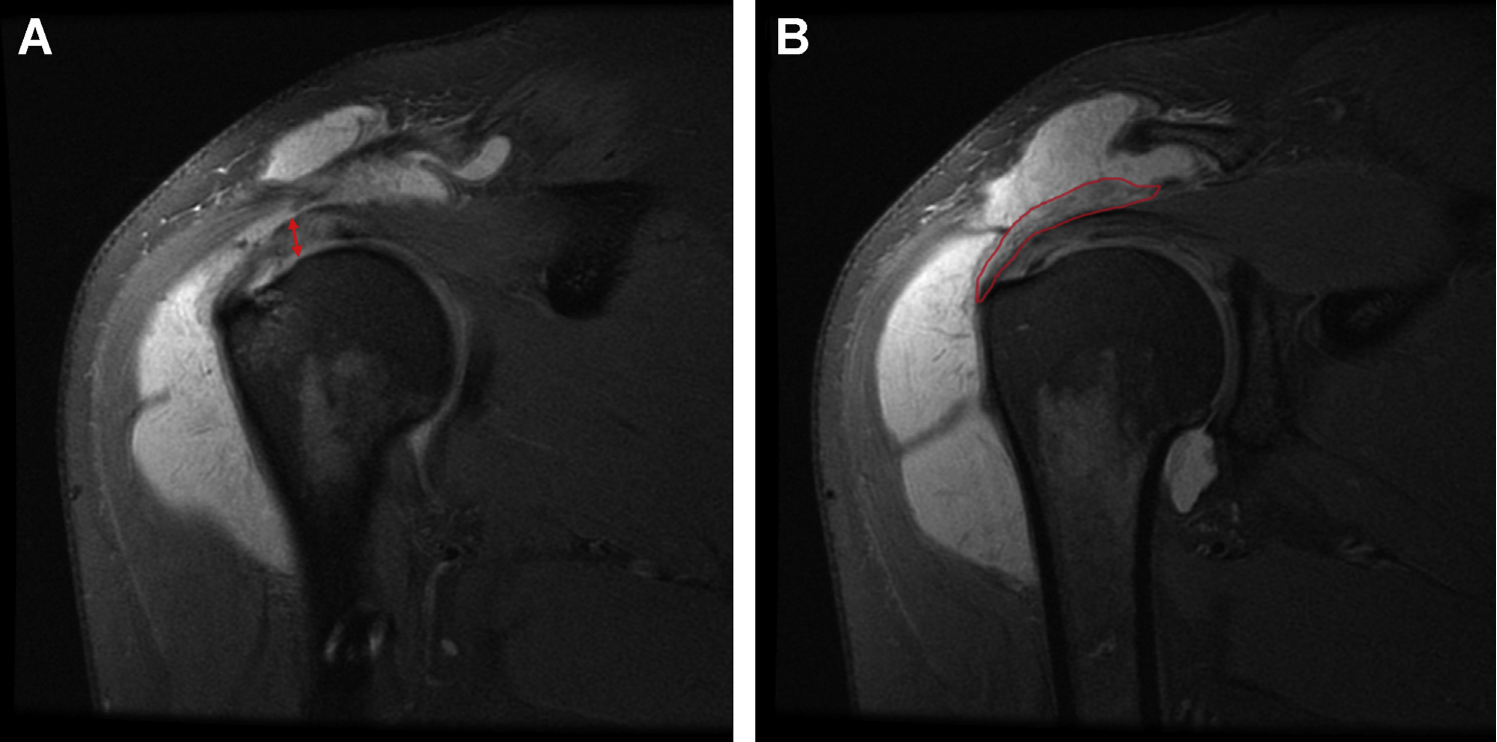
Case two follow-up proton dense fat-saturated MRI coronal sections revealing massive subacromial and subdeltoid fluid. Also noted is improvement in rotator cuff thickness compared to preoperatively (), as well as continued adhesion of the graft to the bursal side of the posterosuperior cuff ().
